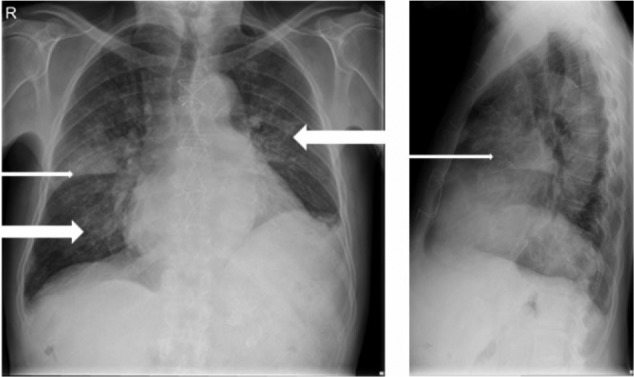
Chest radiography Anteroposterior (left) and sagittal (right). The thin arrows point at the consolidation of the right upper lobe and the bold arrows point at the redistribution.
